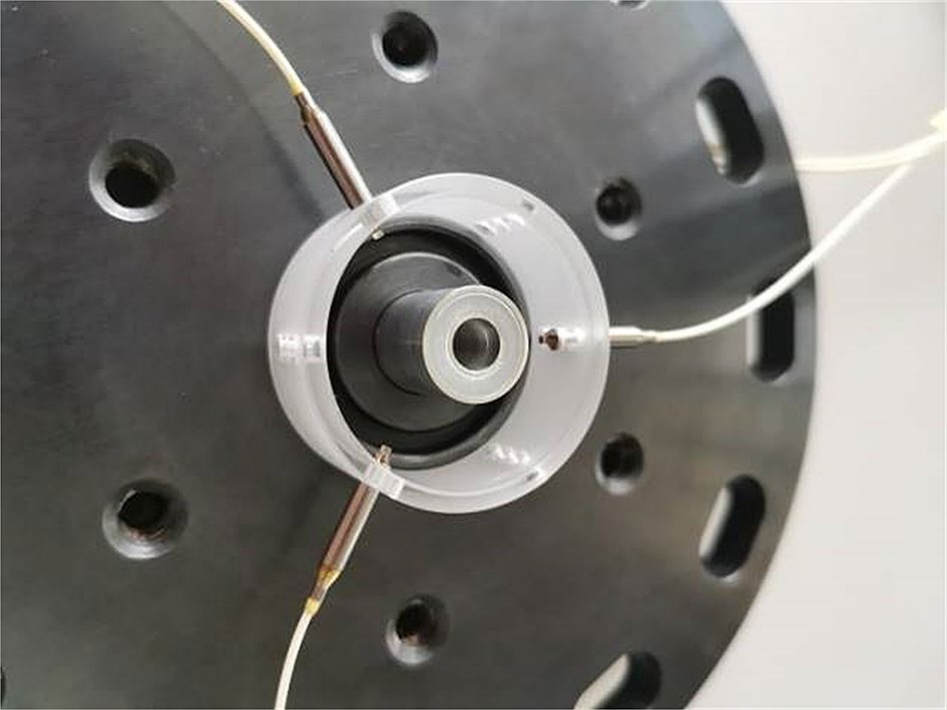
Assembled liners and external photonic Doppler velocimetry probes.
